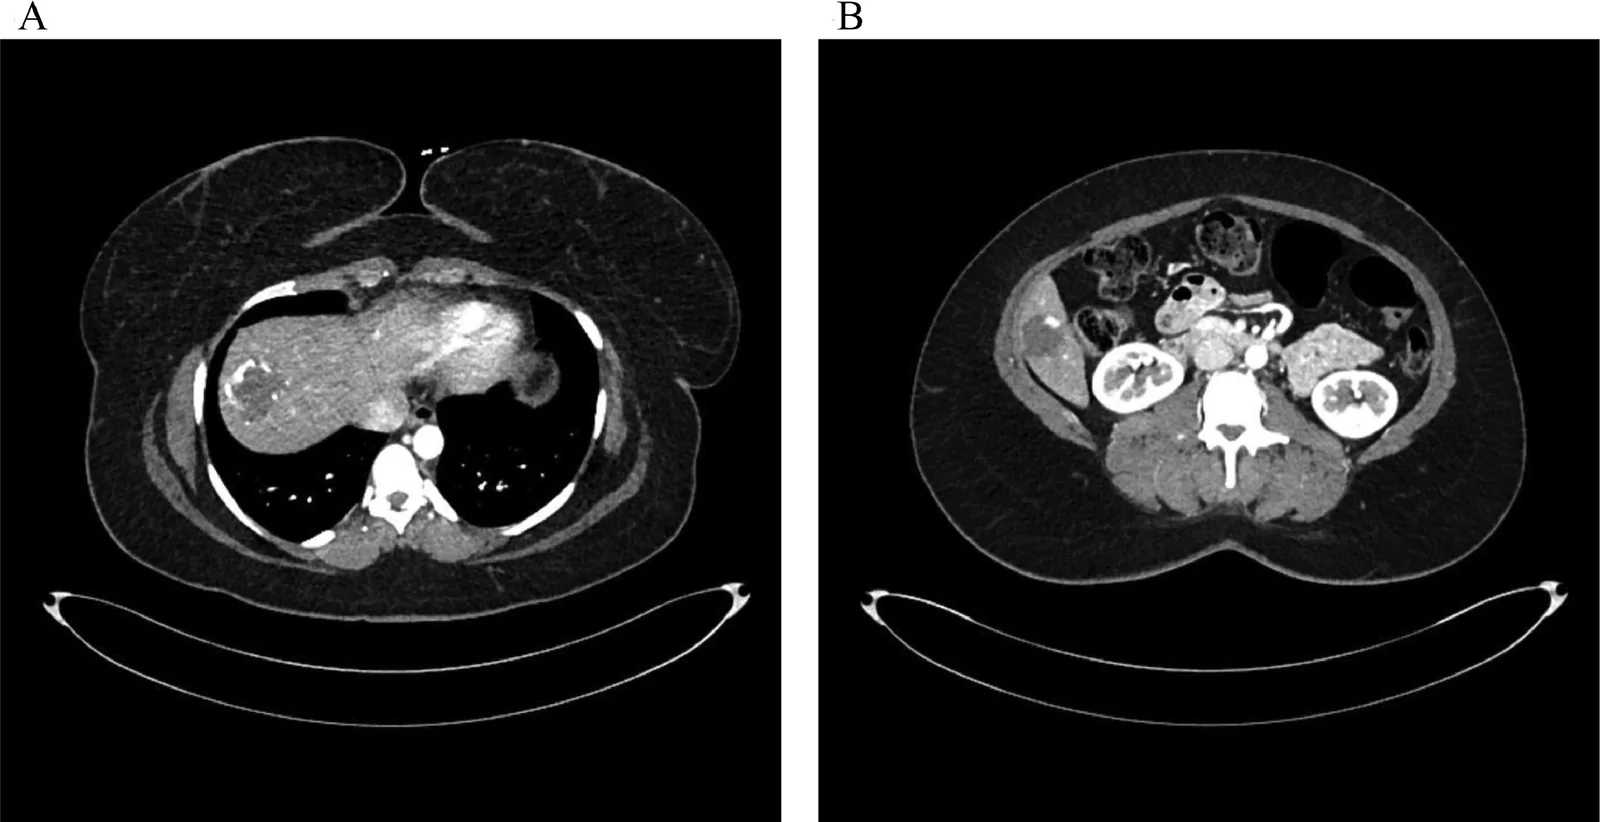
Axial CT findings of multifocal hepatic hemangiomas after a single session of embolization. (A) CT abdomen multi-phase W contrast demonstrates a 3.9 cm × 3.6 cm lesion on the axial view (craniocaudal diameter 3.8 cm, measured on sagittal view; not shown) in segment 7/8 with nodular, discontinuous peripheral enhancement, likely representing a hemangioma status post treatment changes. Pretreatment lesion measured 5.1 × 5.9 cm × 6.4 cm. (B) CT abdomen multi-phase W contrast demonstrates a 2.3 cm × 2.4 cm lesion on the axial view (craniocaudal diameter 2.7 cm, measured on sagittal view; not shown) in segment 6 with nodular, discontinuous peripheral enhancement, likely representing a hemangioma status post treatment changes. Pretreatment lesion measured 3.0 × 3.5 cm × 3.4 cm.
